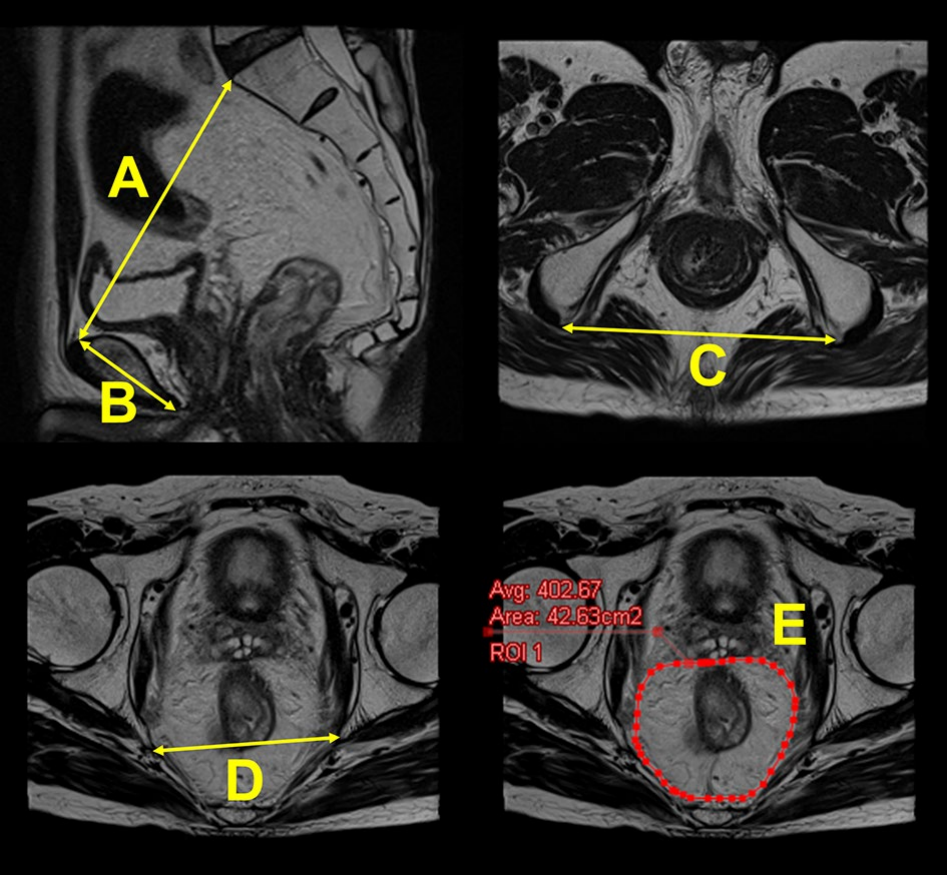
Magnetic resonance imaging pelvimetry. (A) Pelvic inlet (distance between the sacral promontory and superior aspect of the pubic symphysis). (B) Pubic tubercle height (distance from the superior to the inferior middle aspect of the pubic symphysis). (C) Intertuberous distance (distance between the lowest points of the ischial tuberosities). (D) Interspinous distance (distance between the tips of the ischial spines). (E) Mesorectal fat area at the level of the tips of the ischial spines (red circle).
